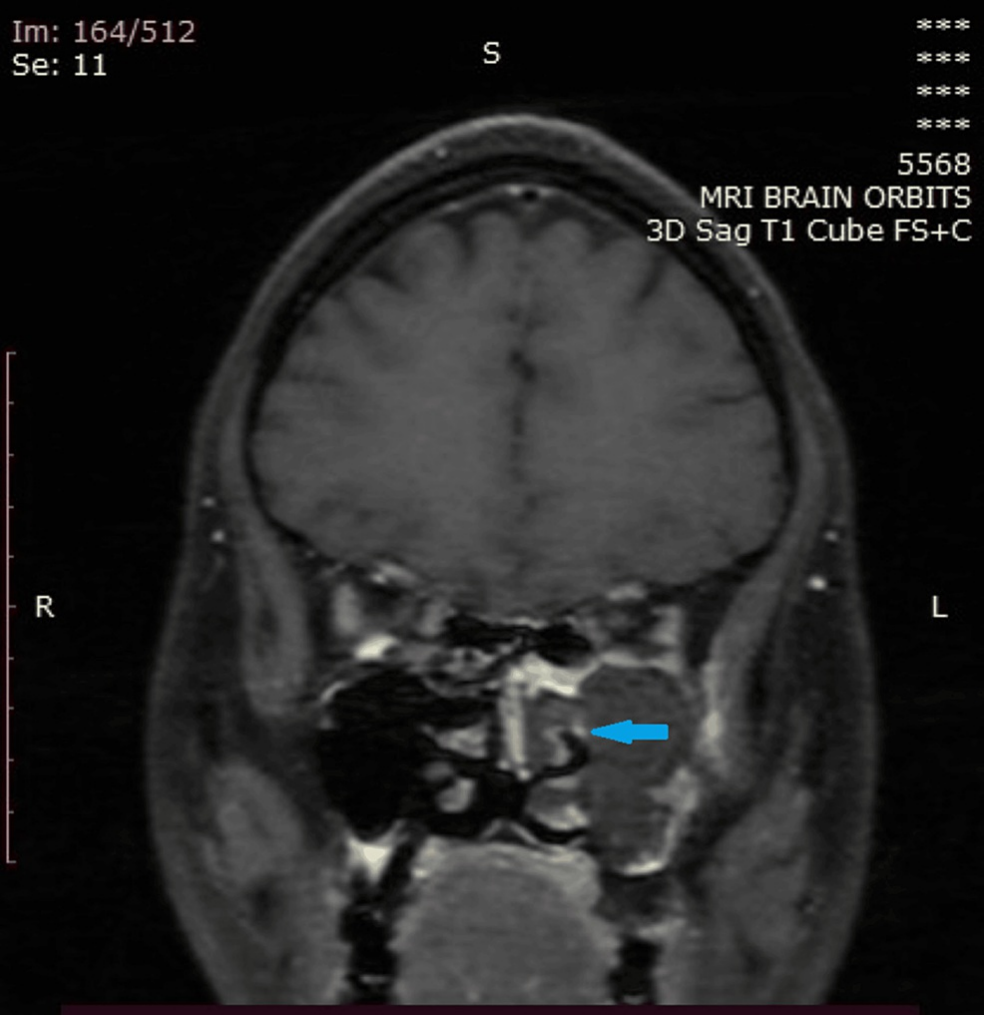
Sinonasal involvement Postcontrast T1-weighted fat-saturated coronal MR image shows non-enhancement of the middle and inferior turbinate left nasal cavity, showing a black turbinate sign and also being associated with left-sided maxillary sinusitis.
